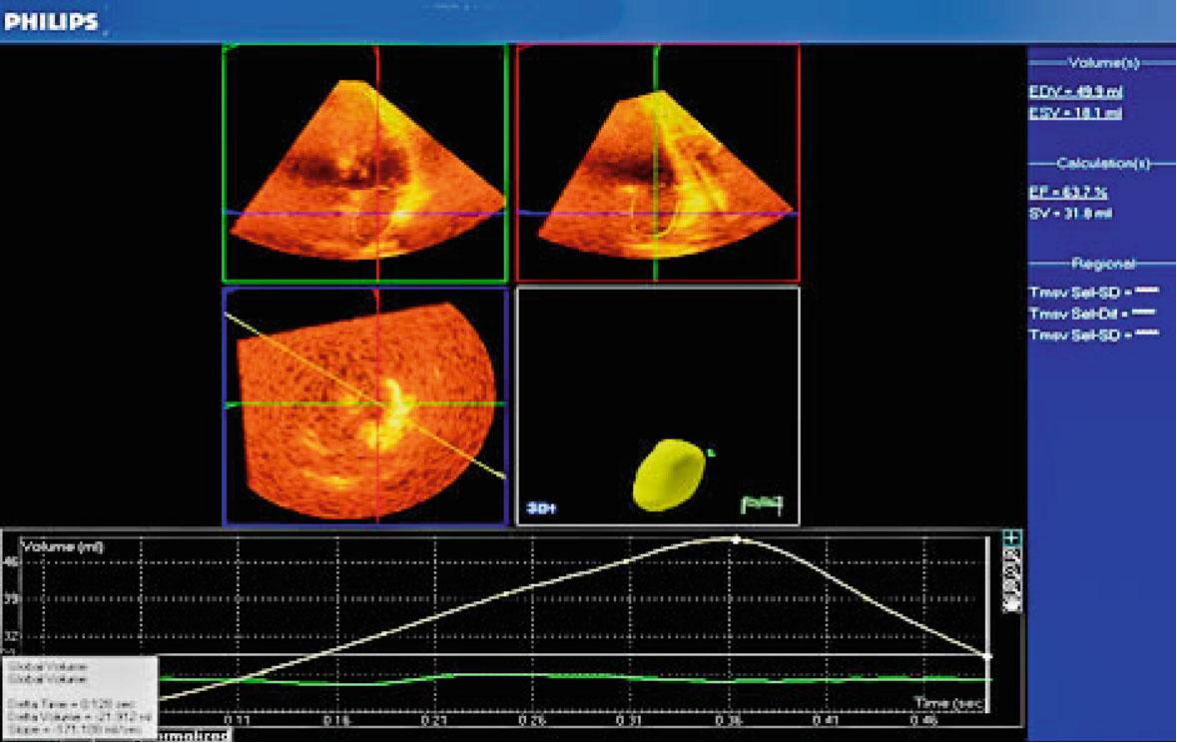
Left atrial volume by real-time three-dimensional echocardiography at last frame before mitral reopening (*V*_preA_).
